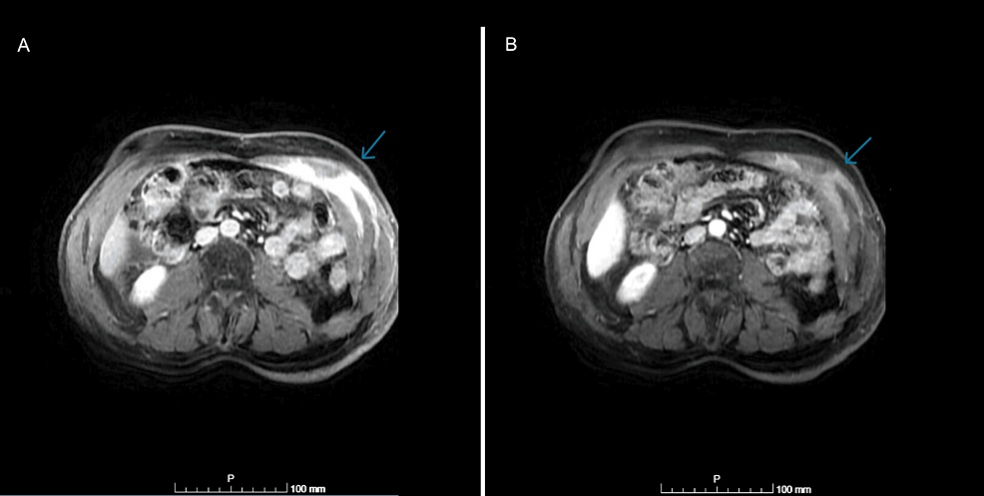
Repeat MRI performed in 2019 showing a thickness in the anterior left abdominal wall MRI: magnetic resonance imaging
